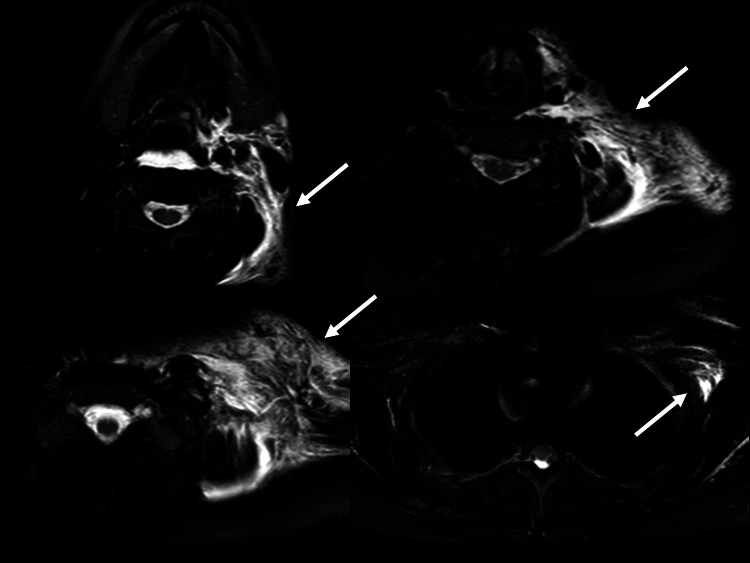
Neck magnetic resonance imaging on arrival Short T1 inversion recovery imaging showed left-side-limited edema (arrow).
